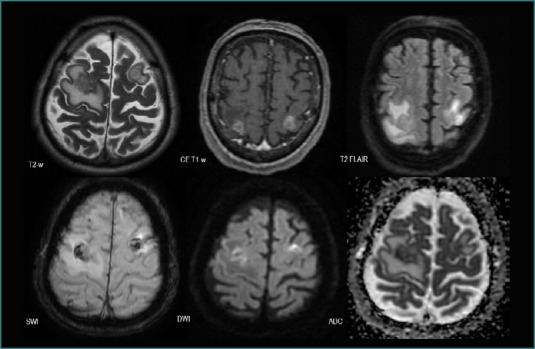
MRI brain acquisition of an NSCLC patient (adenocarcinoma)
